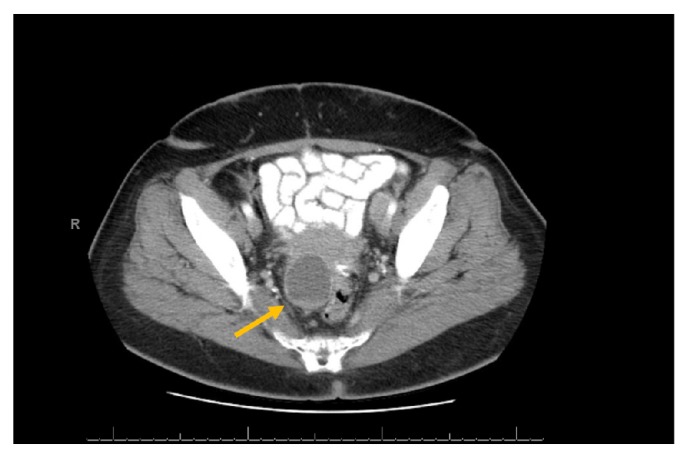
Sagittal image from CT scan following the intravenous administration of 100mL of Omnipaque 350 and 32 ounces oral Omnipaque 350 demonstrating simple paravaginal cystic structure with fluid density contents.
